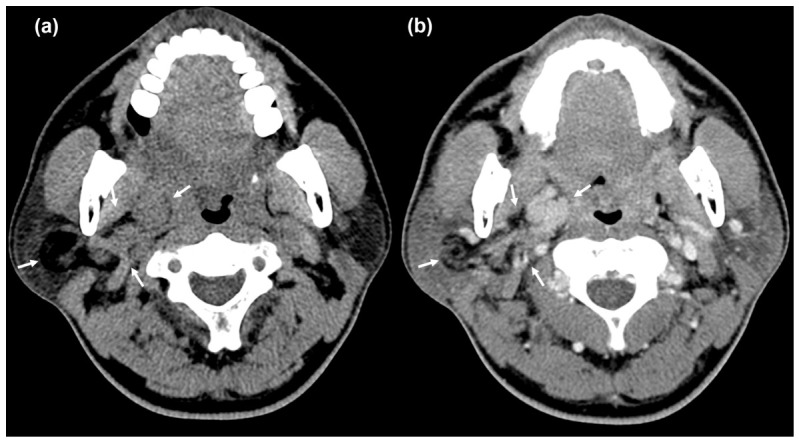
CT findings. (a) Axial non-contrast CT shows a well-defined fat-containing mass centered in the right parotid gland, arising from the deep lobe with lateral extension toward the deep portion of the superficial lobe, and showing medial exophytic extension into the right parapharyngeal space. (b) Axial contrast-enhanced CT shows heterogeneous enhancement with a discrete enhancing solid component along the medial aspect (arrows).
